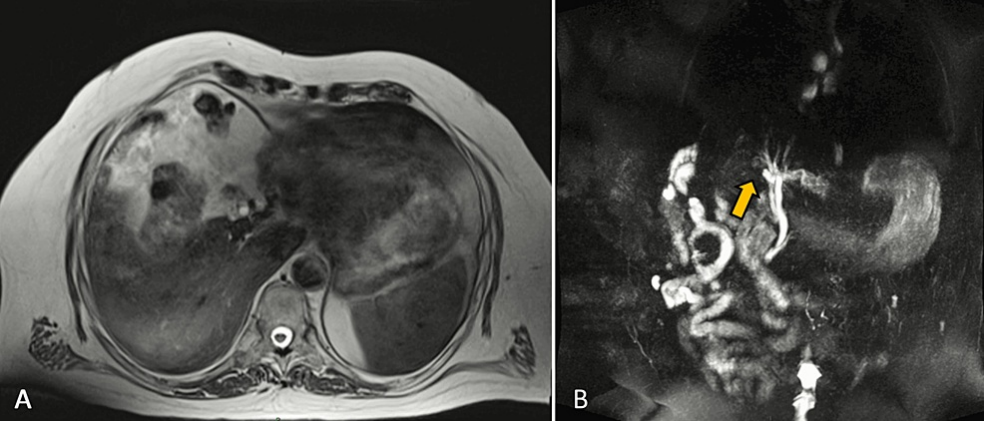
(A) MRI transversal plane with no presence of gallbladder. (B) MRI coronal plane with confirmation of gallbladder agenesis (yellow arrow).
